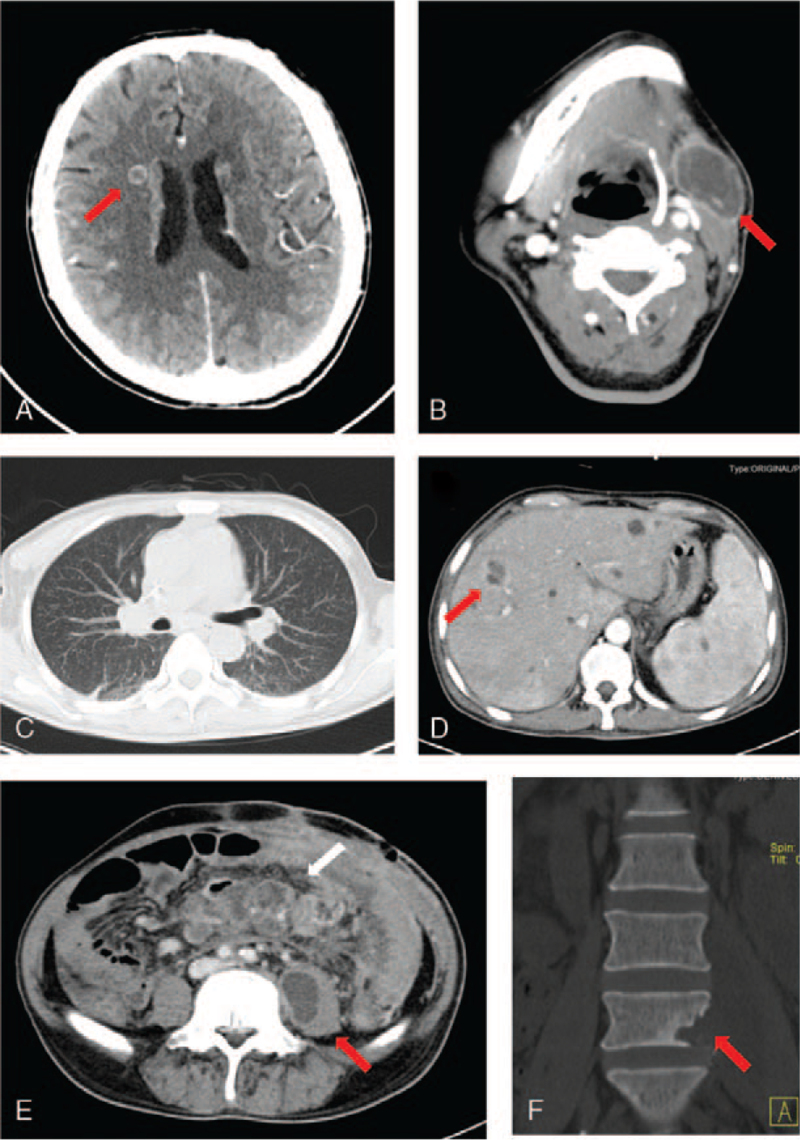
Computer tomography findings of multiple organs in this patient. Head and neck CT showing a strengthening nodule near the right lateral ventricle (A, arrow) and a mass shadow with annular enhancement under the left jaw (B, arrow). Chest computed tomography (CT) showing diffuse miliary nodules in both lungs (C). Abdominal CT reveals multiple ring-enhancing low-density nodules in the liver (D, arrow). Multiple abdominal lymph nodes, including mesenteric lymph nodes, are enlarged with an enhancing rim (E, white arrow). The left psoas major muscle is swollen with a low-density shadow and annular enhancement (E, red arrow). The left side of the L5 vertebra shows bone destruction (F).
